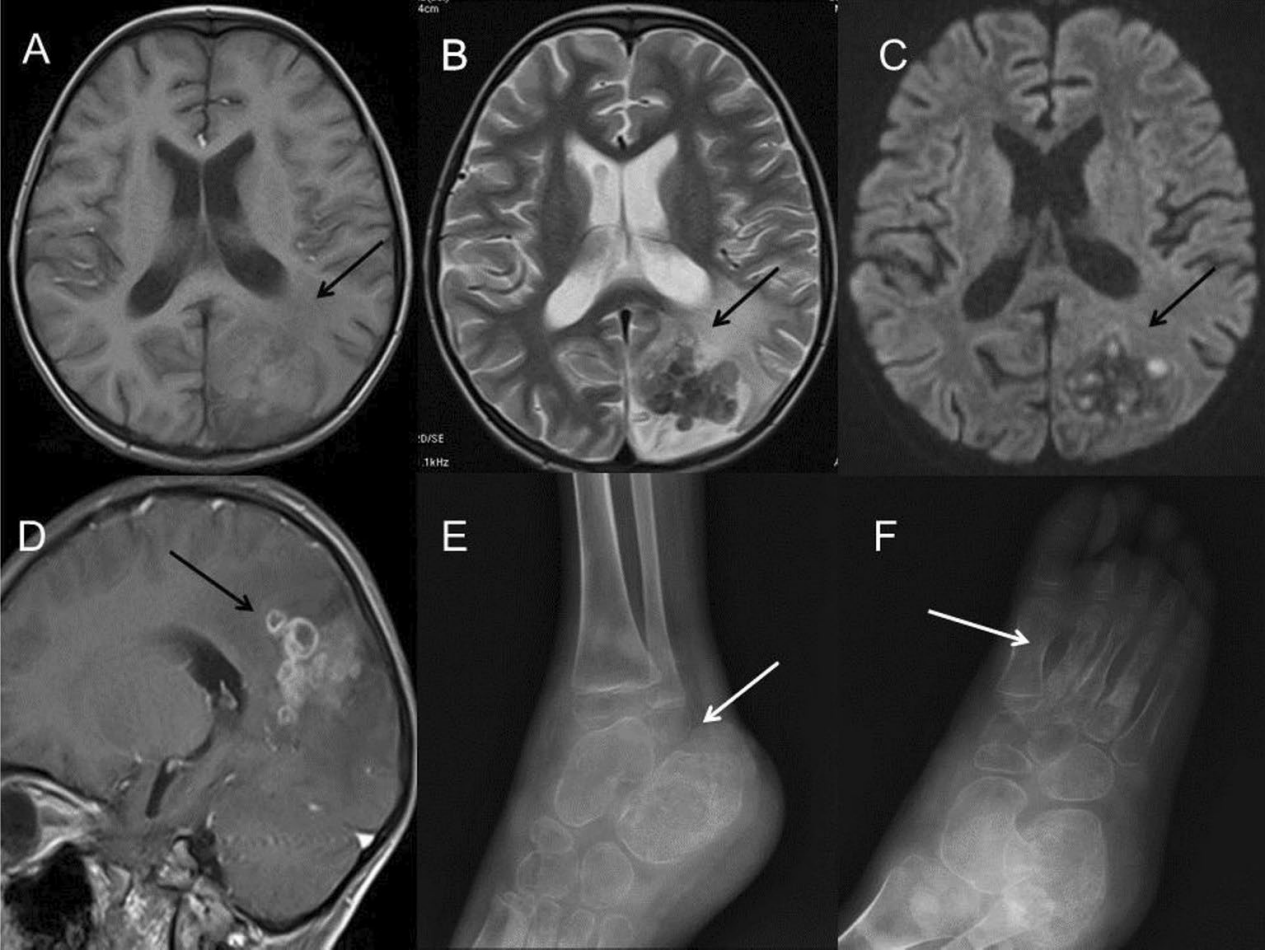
A 5-year-old boy with infection of TB. (A–D) MRI showed multiple small cerebral abscesses (long arrow) in the left parietal and occipital lobes, which had high intensity on T1WI (A), low intensity on T2WI (B), high intensity on DWI sequence (C) and annular enhancement on post-contrast T1WI (D), suggesting the presence of caseous necrosis. Extensive cerebral edema could be found around the lesions. (E,F) Osteomyelitis in the right tibia and foot was found in the same patient, demonstrating local bone erosions and osteosclerosis in the lower metaphysis of the tibia, the calcaneal and the 2nd–4th metatarsal bones (long arrow). Periosteal thickening along the metatarsal bones and swelling soft tissue of the right ankle and foot were found.
